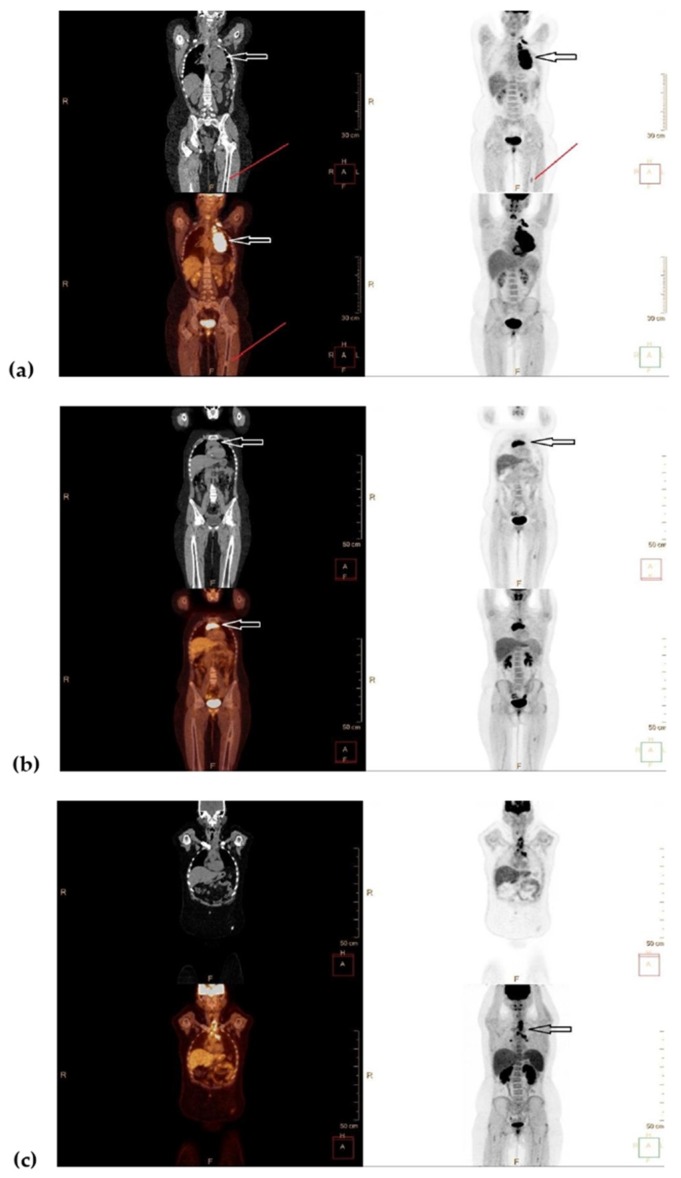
Whole body ^18^FDG PET/CT. (a) At diagnosis. Left supraclavicular and mediastinal ^18^FDG-avid lesions (arrows), ^18^FDG-avid lesion in the left femur (red line), according to lymphoma staging Lugano IV; (b) Relapse. ^18^FDG-avid relapse in the mediastinum (arrows). According to lymphoma response, Deauville 5b. No metabolic changes in the left femur after the biopsy of enchondroma; (c) One month prior to death. ^18^FDG-avid lesions in neck, mediastinum, and hilar lymph nodes bilaterally. According to lymphoma response, Deauville 4.
